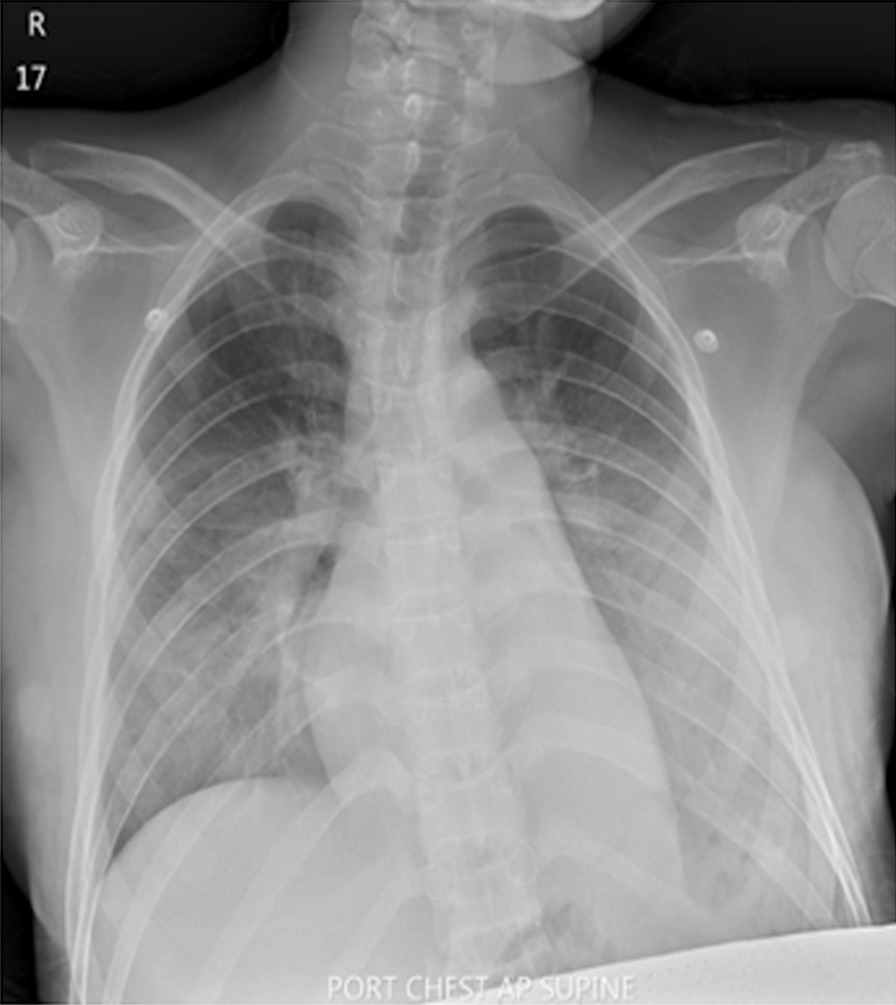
Chest X-ray revealed airspace opacifications with bronchogram presence in the perihilar and lower lung areas bilaterally. No cardiomegaly was demonstrated
